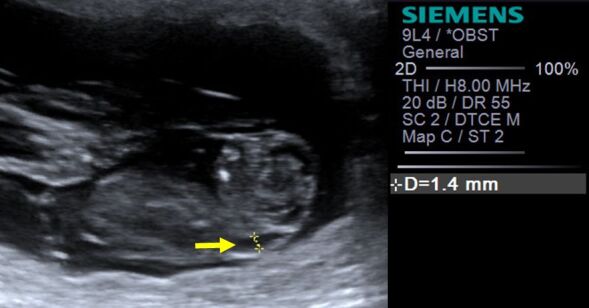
An ultrasonography image of NT (nuchal translucency) measurement (arrow) in a healthy canine fetus (NT = 1.4mm), at 34 days of gestation.
